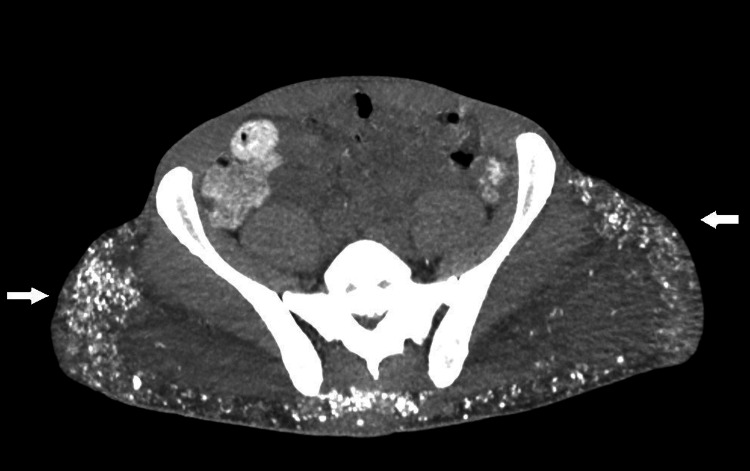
Axial view of a computed tomography image of the pelvis area revealing widespread calcification in the gluteal area bilaterally
